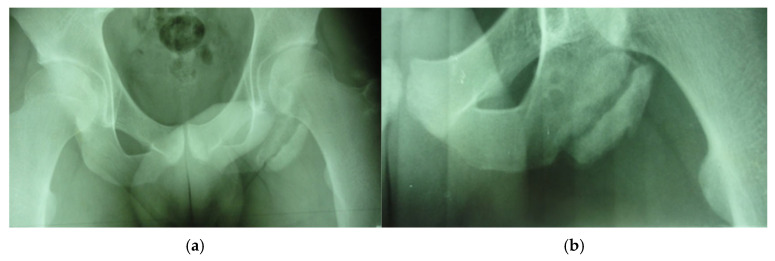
(a) Pelvic X-ray—ischial tuberosity, (b) ischial tuberosity X-ray—detail.
